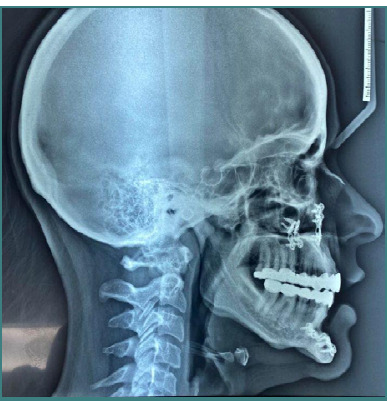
Lateral cephalometric radiograph showing the skeletal and dental structures post-treatment
